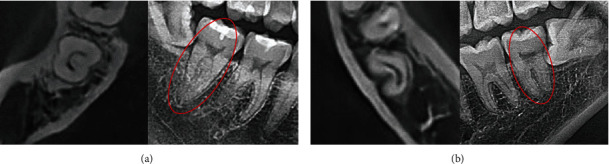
Cone-beam computed tomography (CBCT) scans and panoramic views of teeth observed to have C-shaped canals on CBCT. (a) A case of a mandibular second molar with a fused root on a panoramic radiograph. (b) A case of a mandibular second molar with a separated root on a panoramic radiograph.
